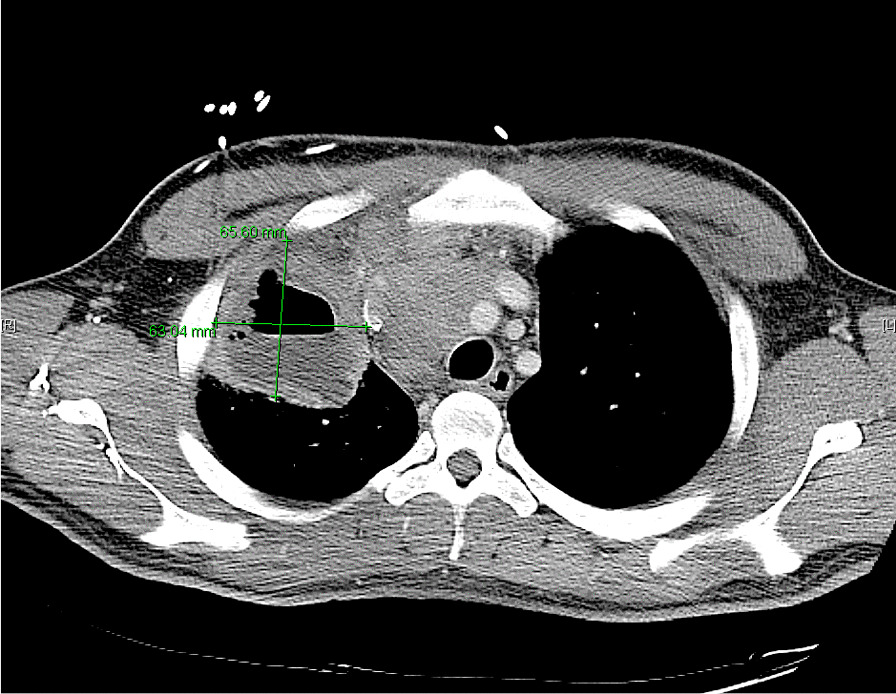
Follow-up CT chest/abdomen/pelvis (axial image). Enlarging right upper lobe cavitary lesion
